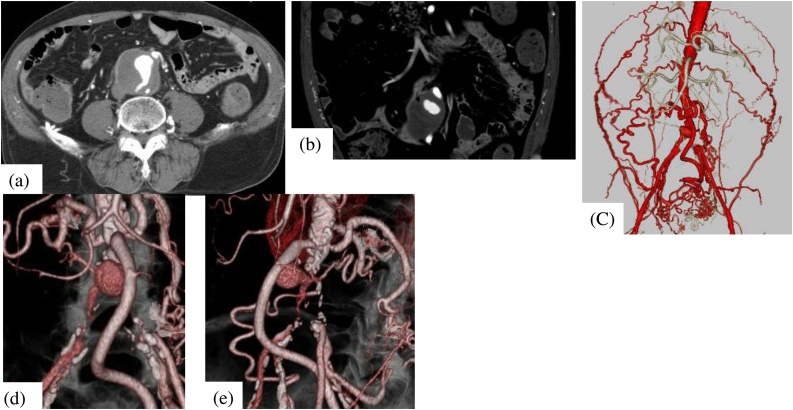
The CT findings at outpatient. (a) On contrast enhanced CT, a true aneurysm was identified near the right side of the terminal aorta. (b) The aneurysm and duodenum were in contact. Air sign was identified within the wall of the aneurysm. (c) Contrast enhanced CT revealed collateral vessels that had developed throughout the body. (d)(e) Contrast enhanced CT revealed the occlusion of the aortic bifurcation. An aneurysm was found near the terminal aorta. The feeding artery of the aneurysm was suspected as right common iliac artery.
